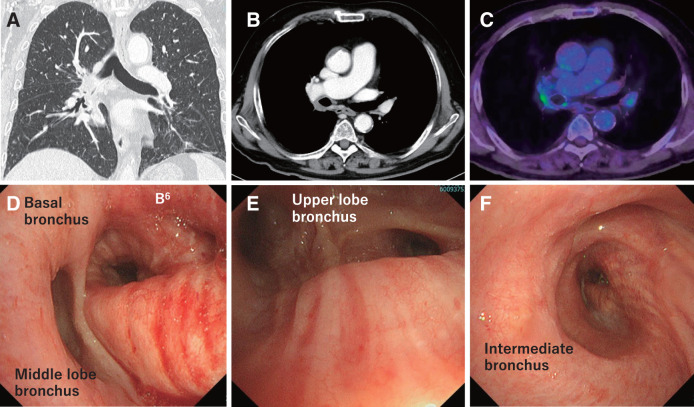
Imaging scan of the patient after chemoimmunotherapy. (A, B) Computed tomography scan showing that the tumor reduced size to 18 mm. (C) PET-CT showed tumor SUVmax was 3.9, decreasing from pre-treatment. (D–F): Bronchoscopy showing the erythema of the bronchial mucosa on the intermediate bronchus and the lower lobar bronchus. PET-CT, positron emission tomography-computed tomography; SUV, standardized uptake value
